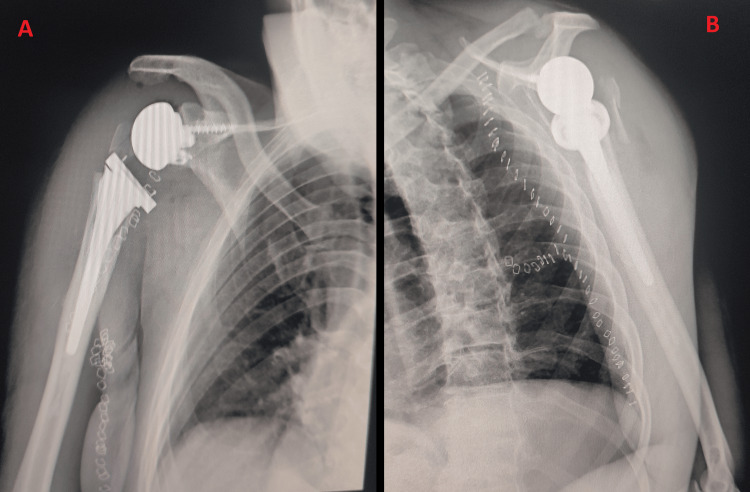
X-ray following reverse total shoulder arthroplasty (RTSA) implantation. A: Anteroposterior view. B: Lateral view.
